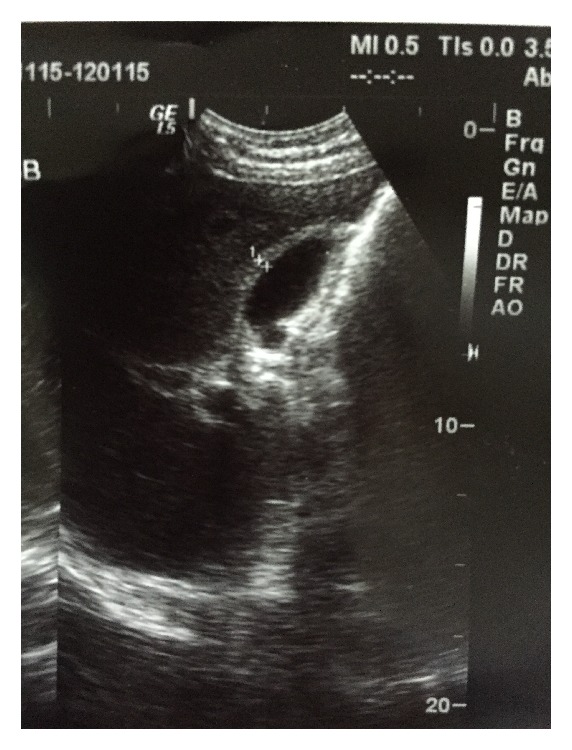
Abdominal ultrasound revealing thickening of the gallbladder wall and absence of stones or dilatation of the biliary tract.
